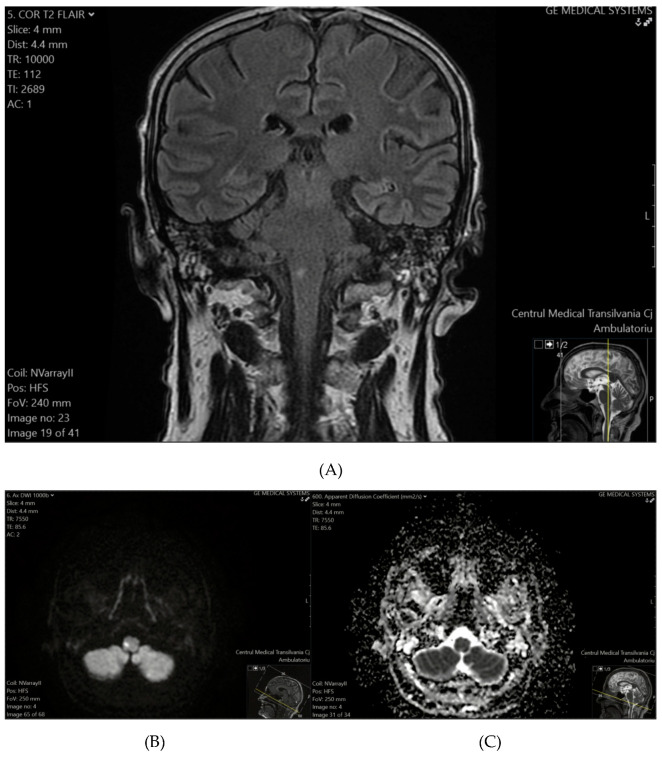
(A) (top) T2-FLAIR sequence reveals a hyperintense focus on the right side of the medulla oblongata. (B) (bottom-left) DWI confirms an ischemic event, demonstrating diffusion restriction with a hyperintense signal. (C) (bottom-right) The corresponding ADC map shows a hypointense signal, further supporting the presence of acute ischemia.
